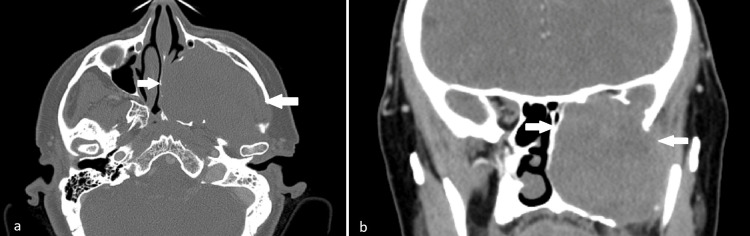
Computed tomography imaging reveals a large sinonasal mass. (a) Axial view; (b) Coronal view
